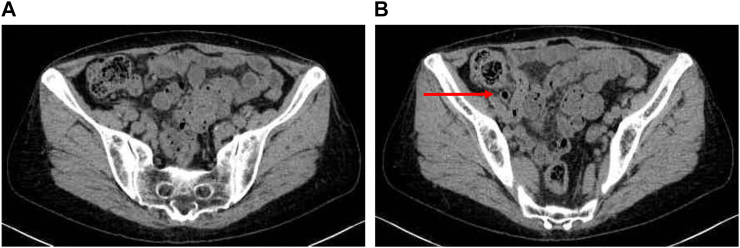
Computed tomography findings. (A) The abscess around the cecum was disappearing. (B) An appendix with gas (red arrow). (For interpretation of the references to colour in this figure legend, the reader is referred to the web version of this article.)
